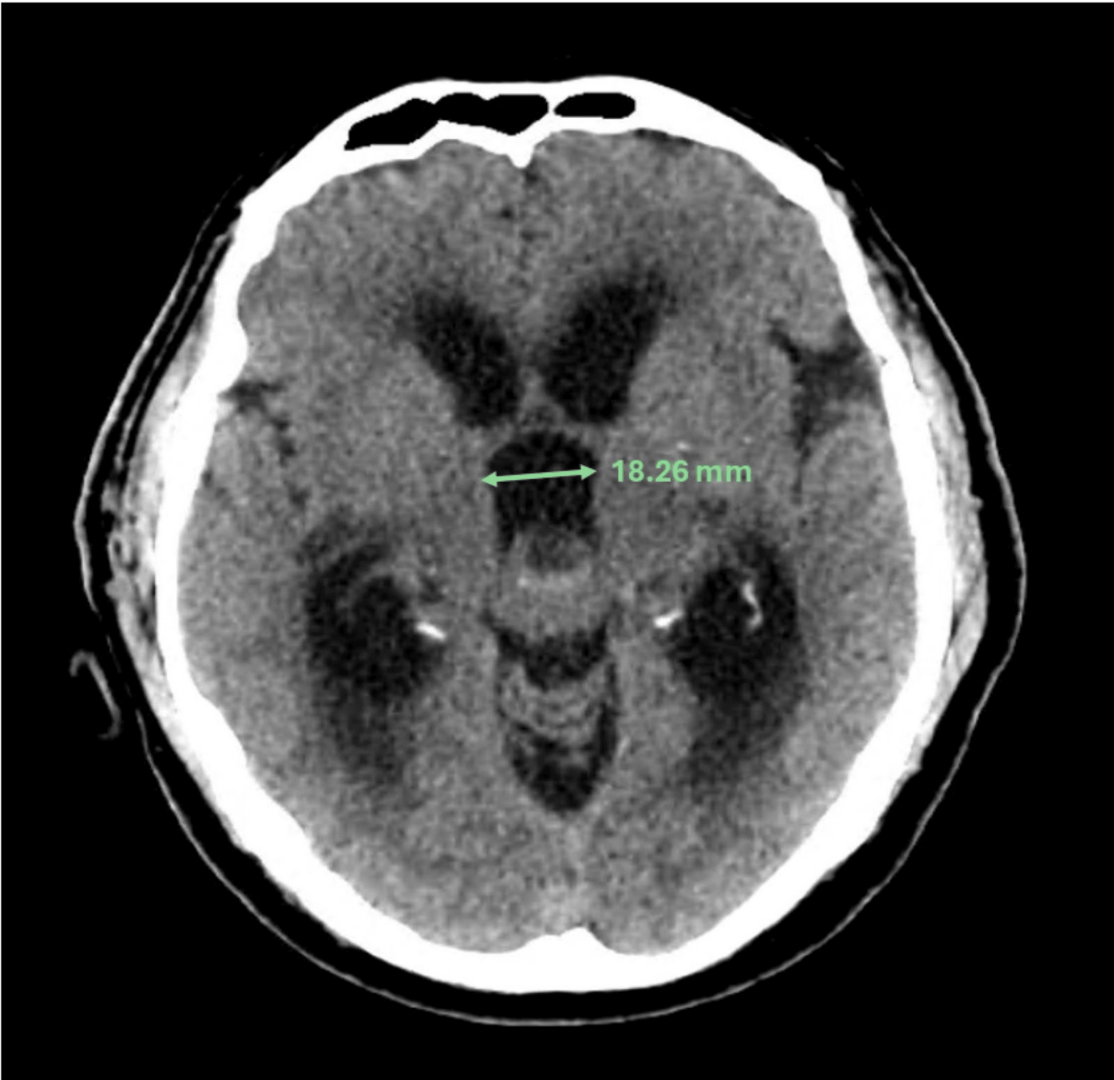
Axial CT head with hydrocephalus / third ventricle dilatation secondary to the aqueduct of Sylvius obstruction by the pineal region mass.
